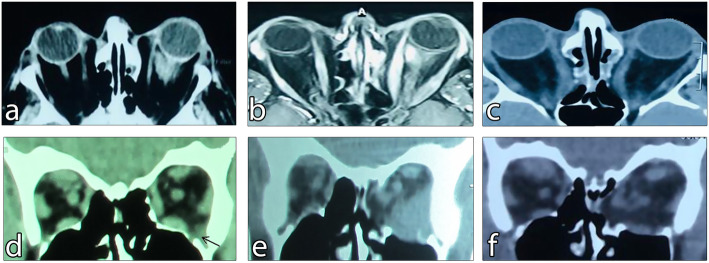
A Baseline CT-scan, revealed an infiltrative mass with intraconal and extraconal expansion in the 1st patient. B Baseline T1- weighted with fat suppression MRI with gadolinium-based contrast agents showed a hyper-intense infiltrative lesion in the left orbit in addition to EOM enlargement in the 1st patient. C Mass resolution was determined in the CT-Scan performed four years after intravenous corticosteroid injection followed by orbital decompression in the 1st patient. D Baseline CT-scan revealed a very small infratemporal mass as indicated by the arrow which remained undiagnosed until its progression in the 2nd patient. E six months’ post decompression surgery CT-scan, showed infiltrative mass enlargement in the 2nd patient. F The lesion was resolved after intravenous corticosteroid administration in the 2nd patient.
